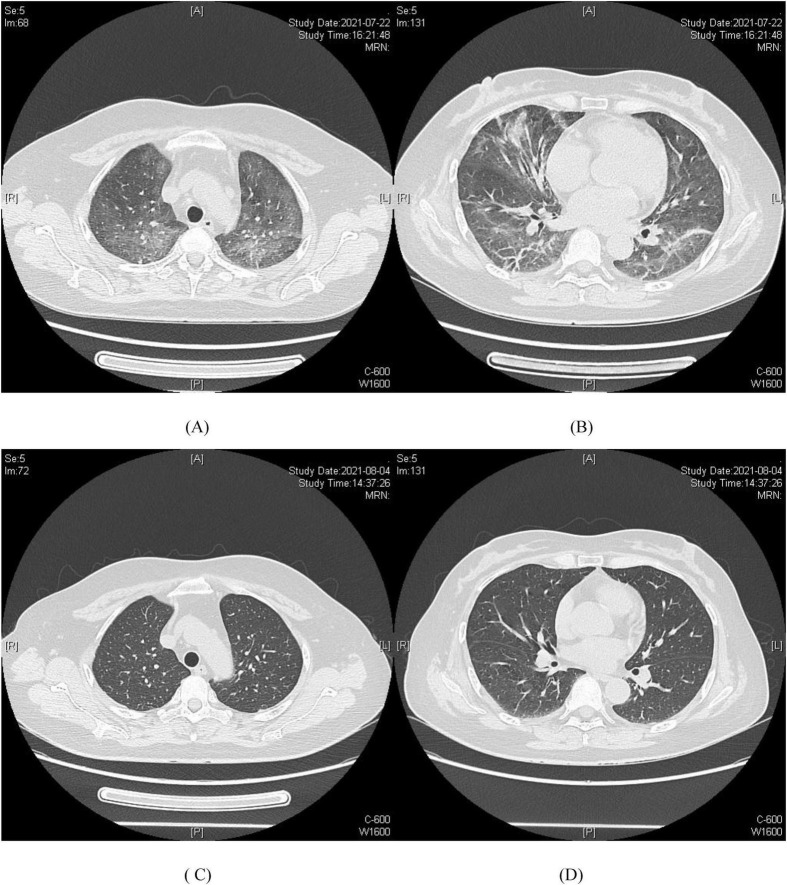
Representative chest CT images of Case 9 before (A,B) and 9 days after (C,D) targeted therapy, showing marked improvement in bilateral patchy infiltrates.
